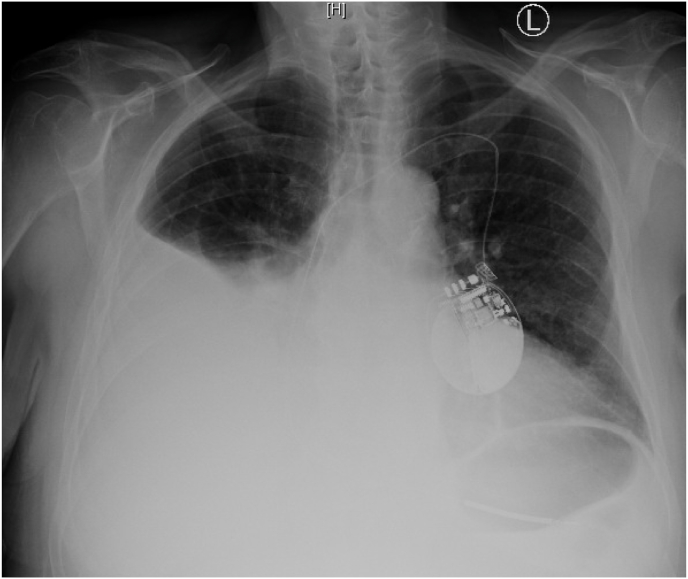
Chest X-ray divulging a large right-sided pleural effusion.
